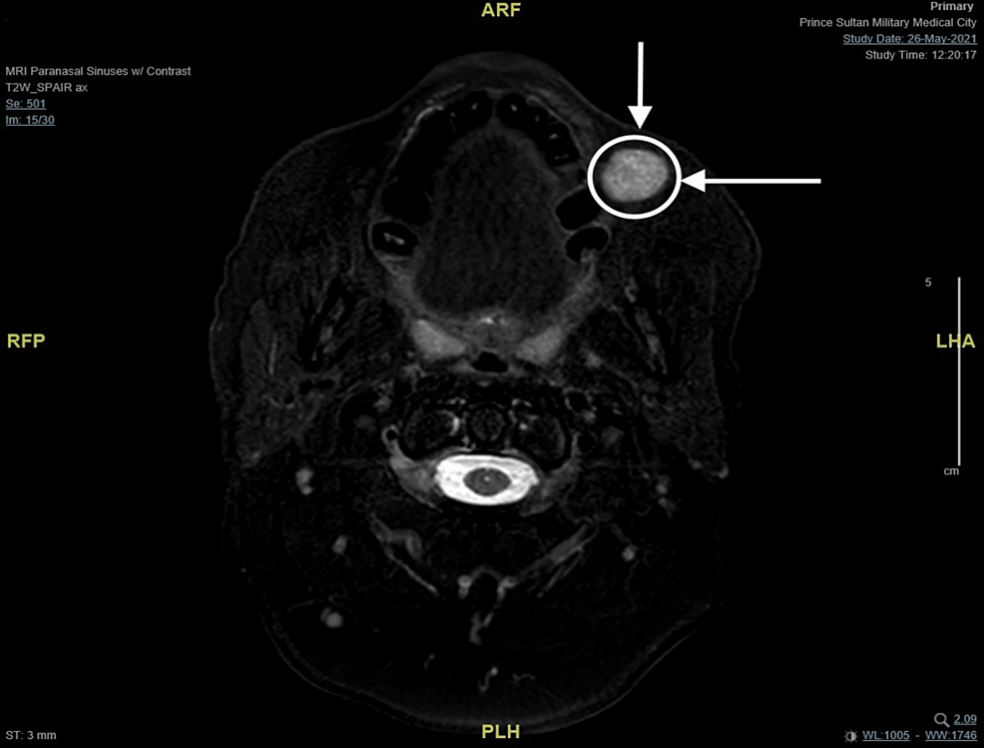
MRI T2-weighted image (axial cut) demonstrating hyperintense mass post contrast on the left buccal space. MRI: magnetic resonance imaging
